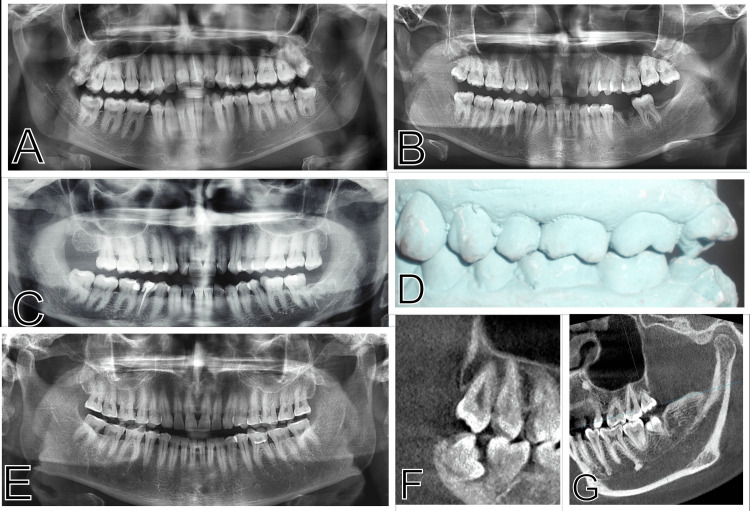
Orthopantomograph, cone beam CT, plaster study model (A) Tilted maxillary third molars in orthopantomograph. (B) Tilted maxillary third molars with supraerupted left maxillary third molar having missing tooth in the lower arch in orthopantomograph. (C) Supraerupted left maxillary third molar having missing tooth in the lower arch in orthopantomograph. (D) Articulated plaster cast with tilted left maxillary third molar with palatal cusp contacting lower tooth. (E) Severe attrition of teeth in orthopantomograph. (F) Distoverted right maxillary third molar in CBCT. (G) Condyle morphology in CBCT.
